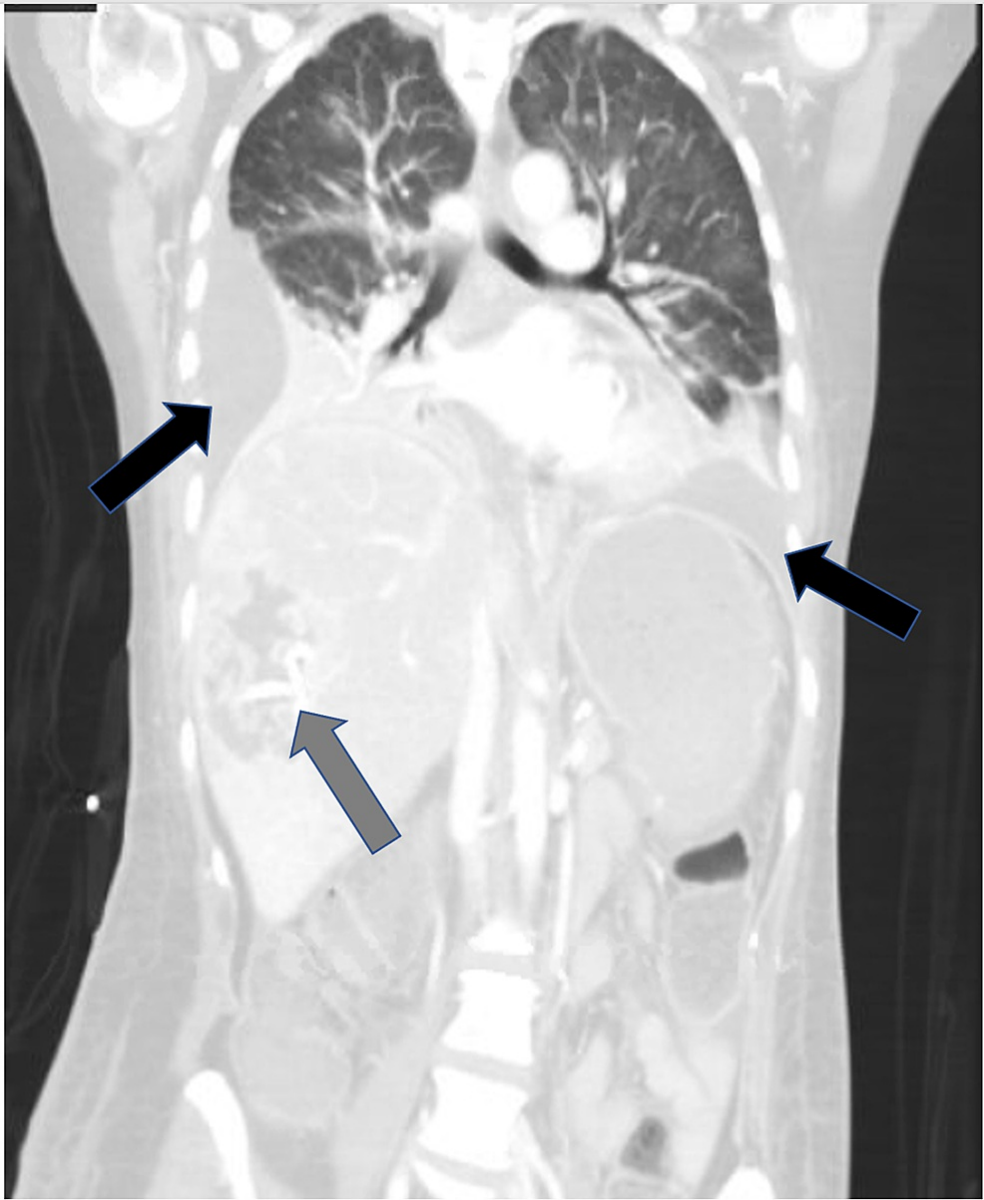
CT chest and abdomen, coronal section, demonstrating bilateral pleural effusions (black arrows), right hemidiaphragm elevation secondary to hepatomegaly with drain (gray arrow) in right hepatic lobe abscess.
